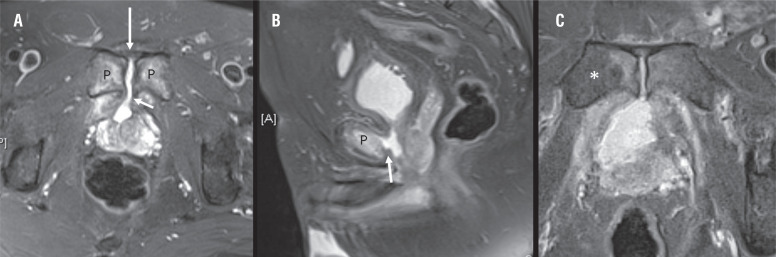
Pelvic MRI with fat suppression technique. (A) Axial T2 - weighted demonstrates the presence of urine (arrow) through the pubic symphysis (P). (B) Sagittal T2 - weighted shows anterior prostate - symphyseal fistula (arrow). (C) Axial T2 - weighted image displays edematous changes within both pubic bones, most markedly on right side (asterisk).
